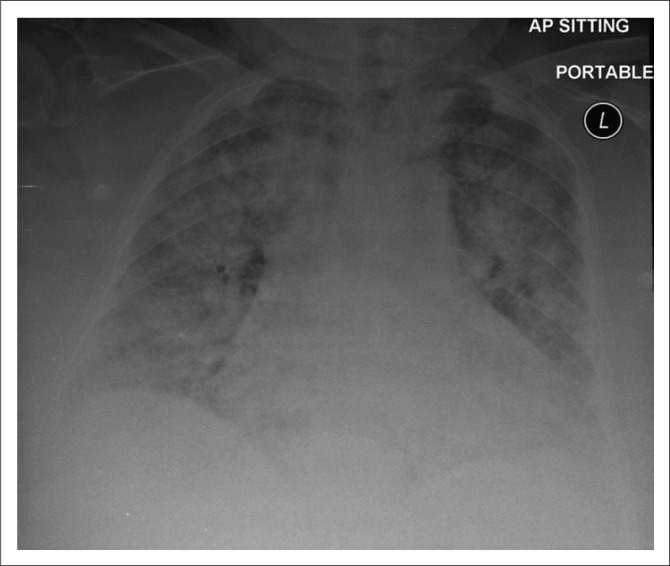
A 69-year-old female admitted to an isolation ward with COVID-19 pneumonia. Portable chest radiograph demonstrates air space opacification with no zonal predominance. *Brixia* score: 18.
